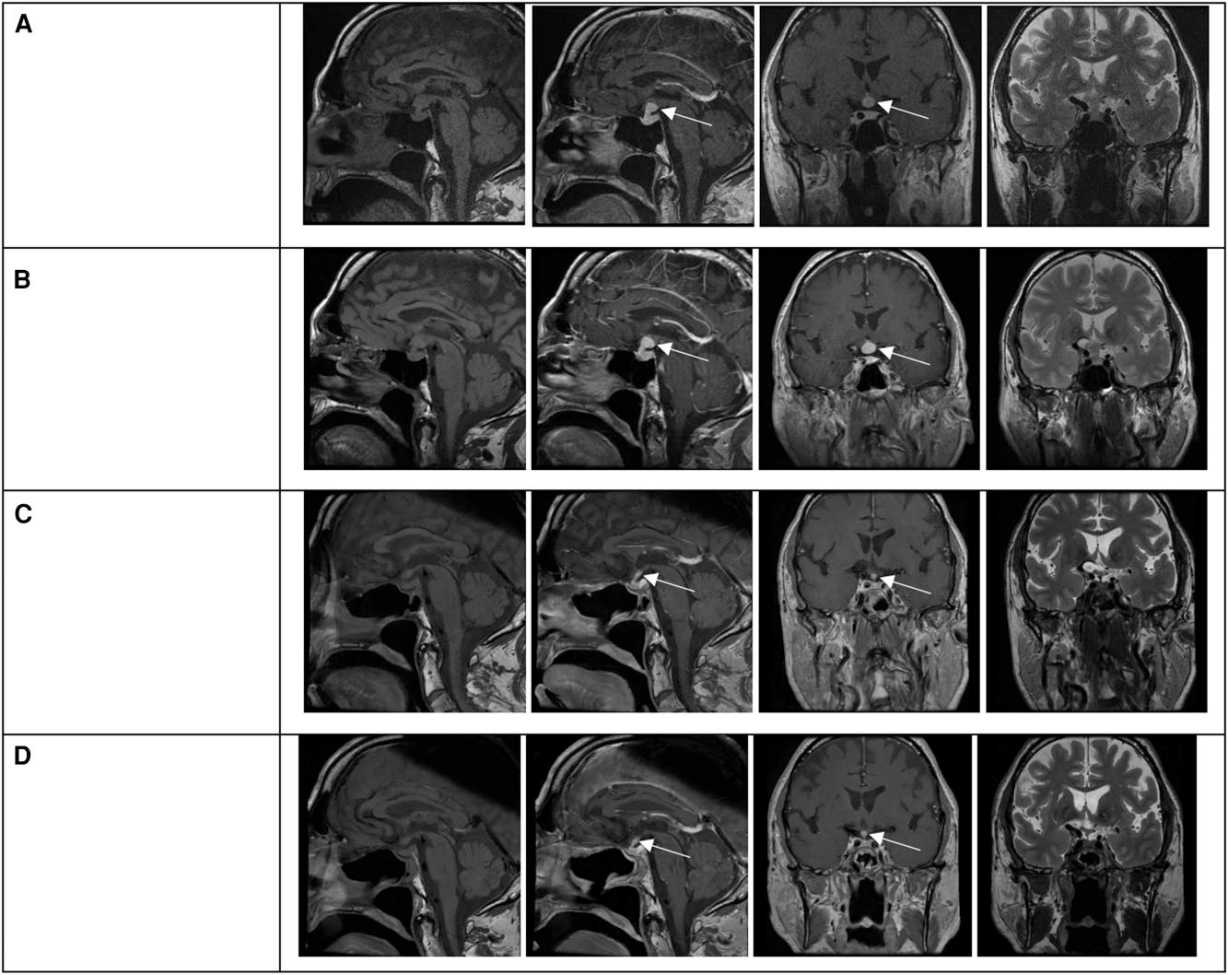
Left to right: noncontrast sagittal T1-weighted, postcontrast sagittal T1-weighted, postcontrast coronal T1-weighted, and noncontrast coronal T2-weighted. White arrows denote the suprasellar lesion in the images where it is best visualized. (A) At presentation August 2022; (B) before biopsy March 2023; (C) 1 month after starting 40 mg/day prednisone August 2023; (D) after 10 months of prednisone 5 mg/day June 2024.
